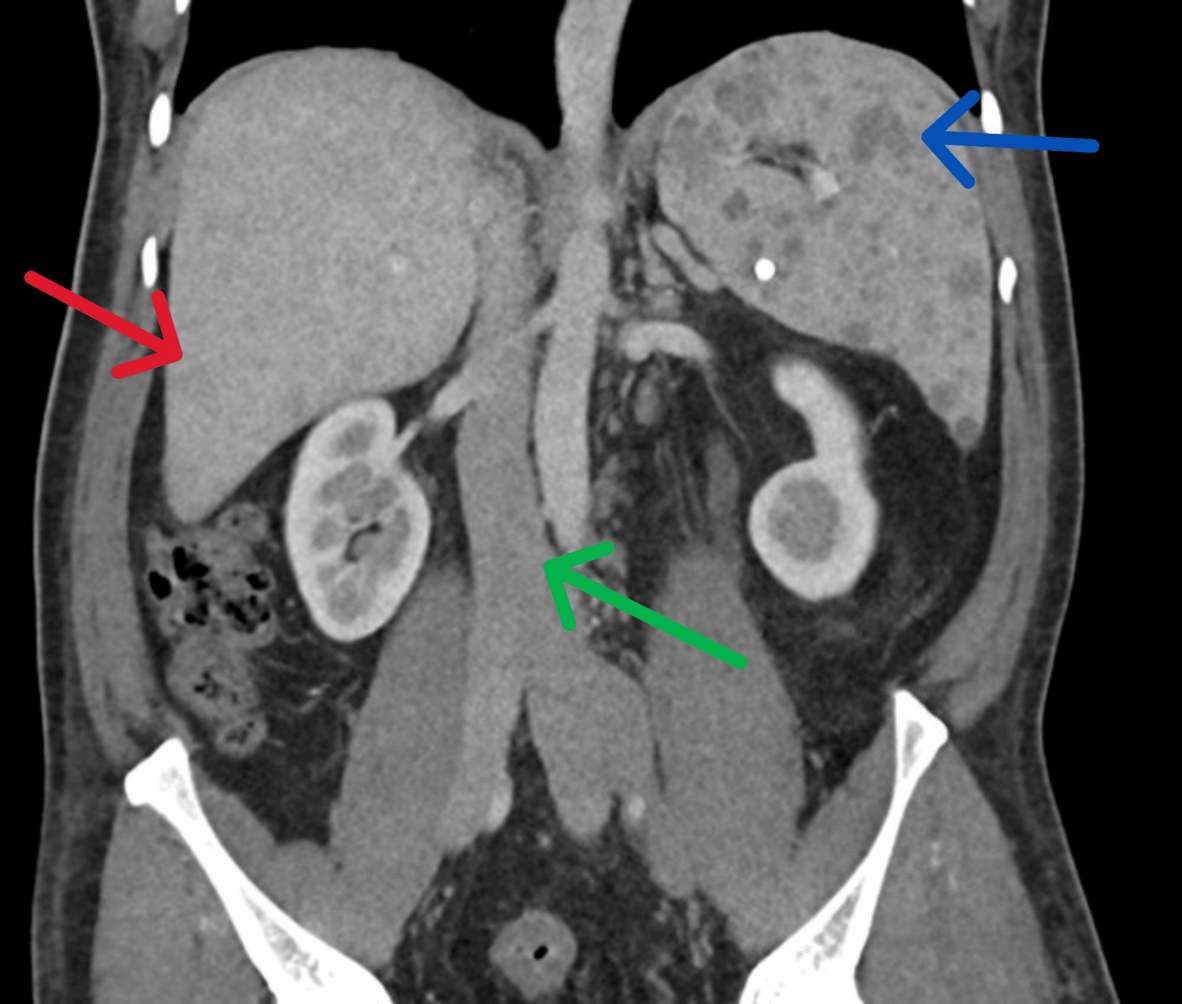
CT abdomen (coronal view) showing innumerable hemangiomas in the liver (red arrow) and spleen (blue arrow), along with an enlarged IVC (green arrow). IVC: inferior vena cava
